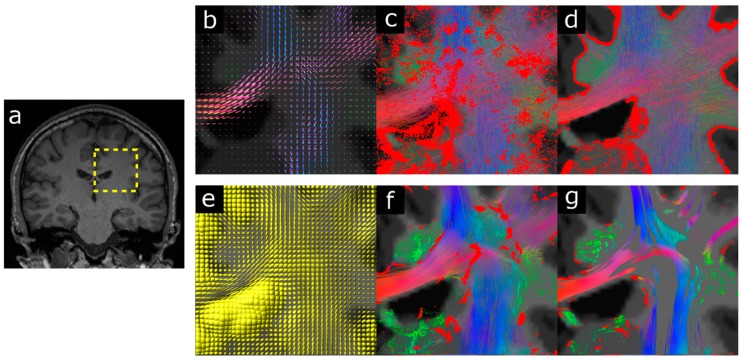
Demonstration of the effects of incorporating anatomical priors during fibre-tracking. (a) Coronal T1-weighted anatomical image highlighting by the yellow dotted lines the zoomed region. (b) Fibre Orientation Distributions on the zoomed region, overlaid on the segmented anatomical image. (c) Streamlines computed using probabilistic tracking without incorporating anatomical priors. (d) Corresponding streamlines from probabilistic tracking when the Anatomically Constrained Tractography (ACT) framework is considered. (e) Diffusion tensors on zoomed region. (f) DTI-based deterministic fibre-tracking results without incorporating anatomical priors. (g) Corresponding DTI-based streamline results when the ACT framework is considered. Streamline terminations are indicated by the red dots. For all cases, only streamlines within a 0.9 coronal slice are shown. Figure modified from a figure previously published in [47], with permission of Elsevier Inc, 2012.
